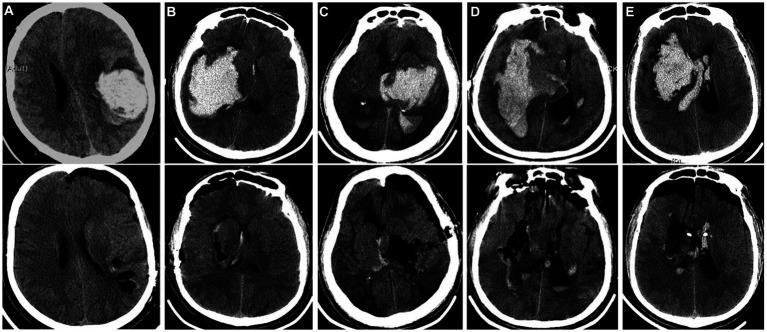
CT comparison of typical cases of hypertensive intracerebral hemorrhage before and after endoscopic clearance. (A): Simple lobar hematoma. The hematoma almost breaks out of the cortex. Bedside CT locates the shallowest part of the hematoma as the puncture point, and a channel was puts to remove the hematoma; (B): The amount of hematoma in the basal ganglia area is large, and the hematoma has reached the temporal lobe cortex. The bedside CT located the shallowest part of the hematoma as the puncture point, and a channel was placed to remove the hematoma; (C): The hematoma in the basal ganglia and thalamus breaks into the ventricles of the brain. The hematoma extends from the cortex to the thalamus. The longest diameter of the hematoma is to clear the hematoma through the temporal lobe fistula. The channel does not need to swing obviously, and the damage is small; (D): The giant fusiform hematoma in the basal ganglia was removed by navigation via the long frontal axis approach; (E): The hematoma in the basal ganglia area broke into the ventricle, and the channel was placed through the puncture point of the ventricle under the guidance of navigation. At the same time, the hematoma in the basal ganglia area and part of the hematoma in the ventricle were cleared.
